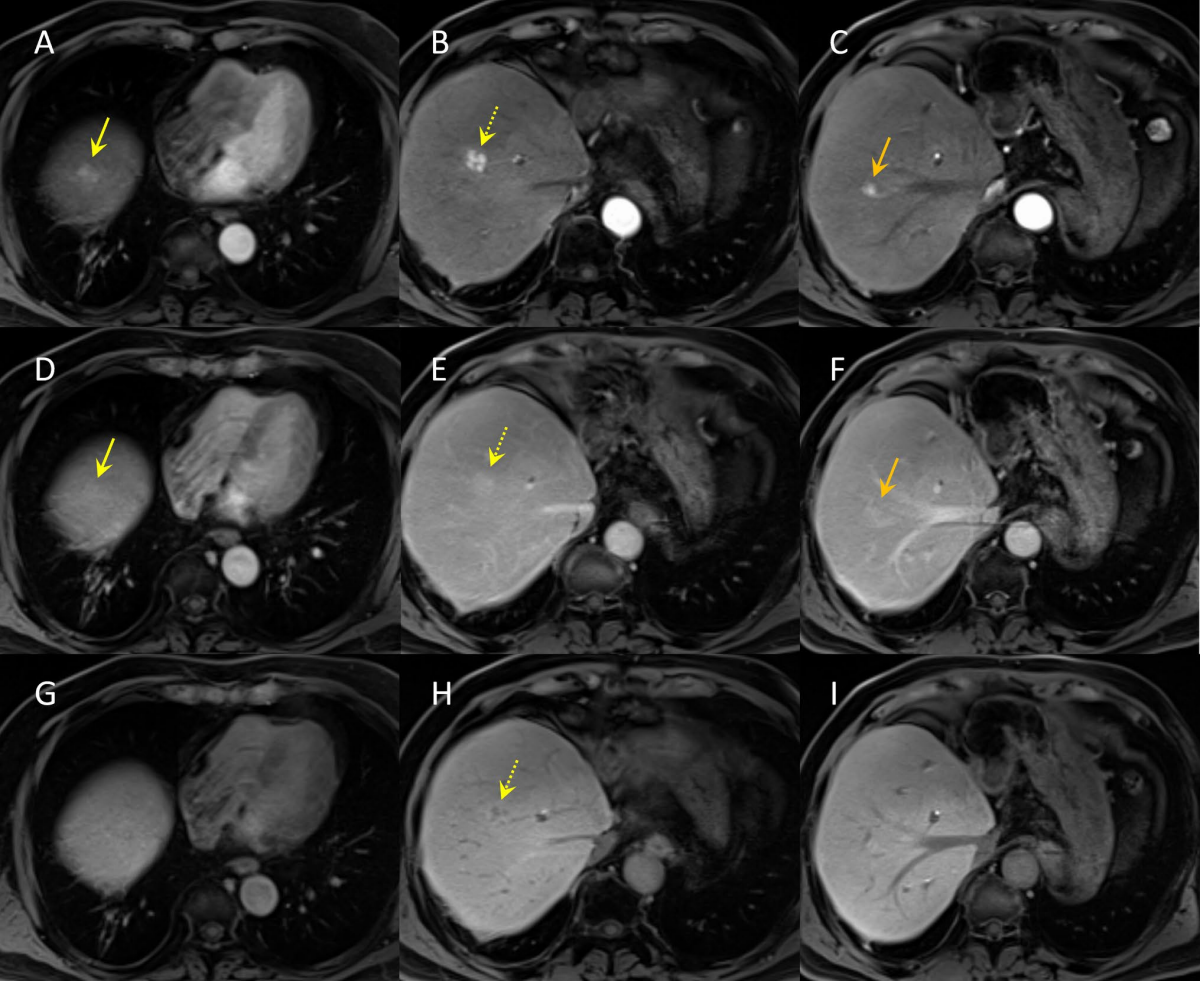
Gadoxetic acid-enhanced magnetic resonance imaging of focal nodular hyperplasia three years after liver transplantation. Three lesions were hyperintense in the arterial phase (A: dome, yellow arrow, B: S8, dotted yellow arrow, C: S8/7, orange arrow). These lesions remained hyperintense in the portal venous phase (D: dome, yellow arrow, E: S8, dotted yellow arrow, F: S8/7, orange arrow). Dome and S8/7 lesions were isointense, but S8 lesion showed hypointensity inside and hyperintensity outside in the hepatobiliary phase (G: dome, yellow arrow, H: S8, dotted yellow arrow, I: S8/7, orange arrow)
